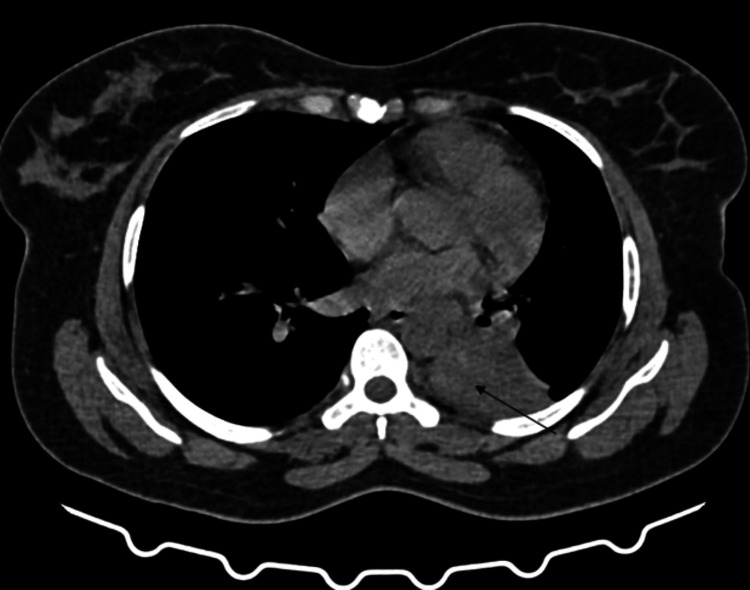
Computed tomography (CT) axial section of the thorax, using the mediastinal window, reveals a soft tissue lesion in the left lower lobe, as indicated by the black arrow.
